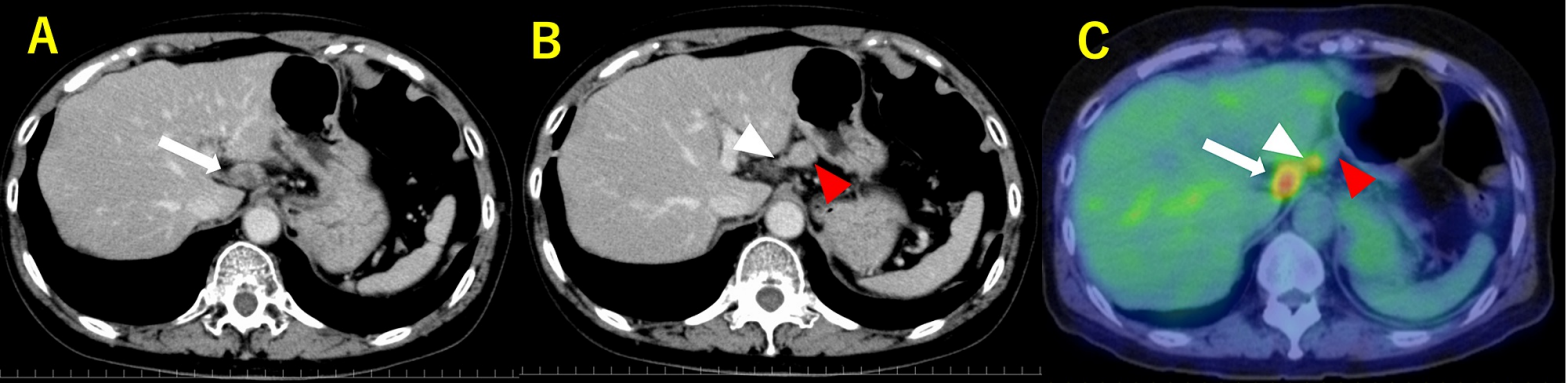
CT and PET/CT Pre-operative enhanced computed tomography (A,B) and Fluorine-18 fluorodeoxyglucose (FDG) positron emission tomography/computed tomography imaging (C) demonstrates a slightly enlarged lymph node with high FDG uptake (SUVmax =5.6, white arrow). The size of the cyst (red arrowhead) was unchanged. FDG uptake (SUVmax =3.9, white arrowhead) showed in stricture region.
